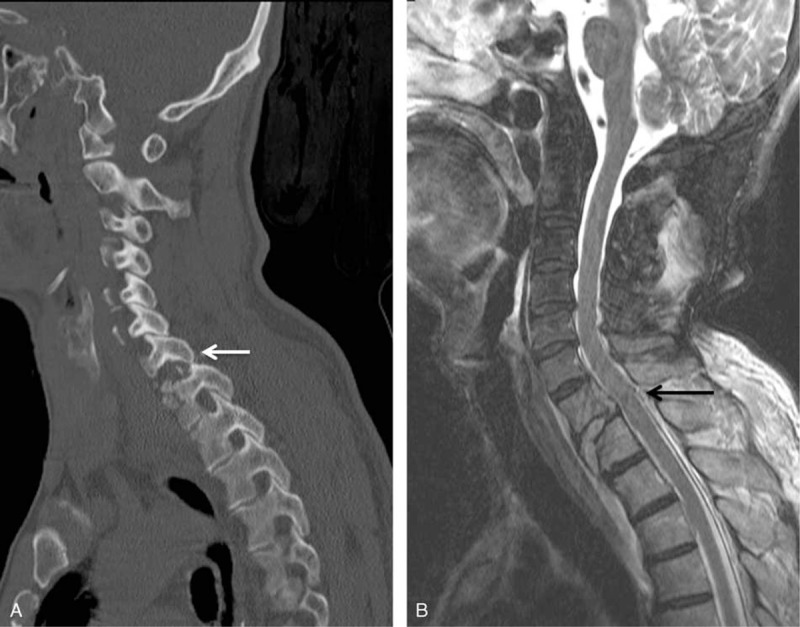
(A) Three-dimensional computed tomography image demonstrating C7/T1 unilateral facet dislocation. (B) Magnetic resonance image demonstrating spinal cord contusion and hemorrhage. There were ruptured disc materials on C7/T1 and rupture of the anterior longitudinal ligament.
